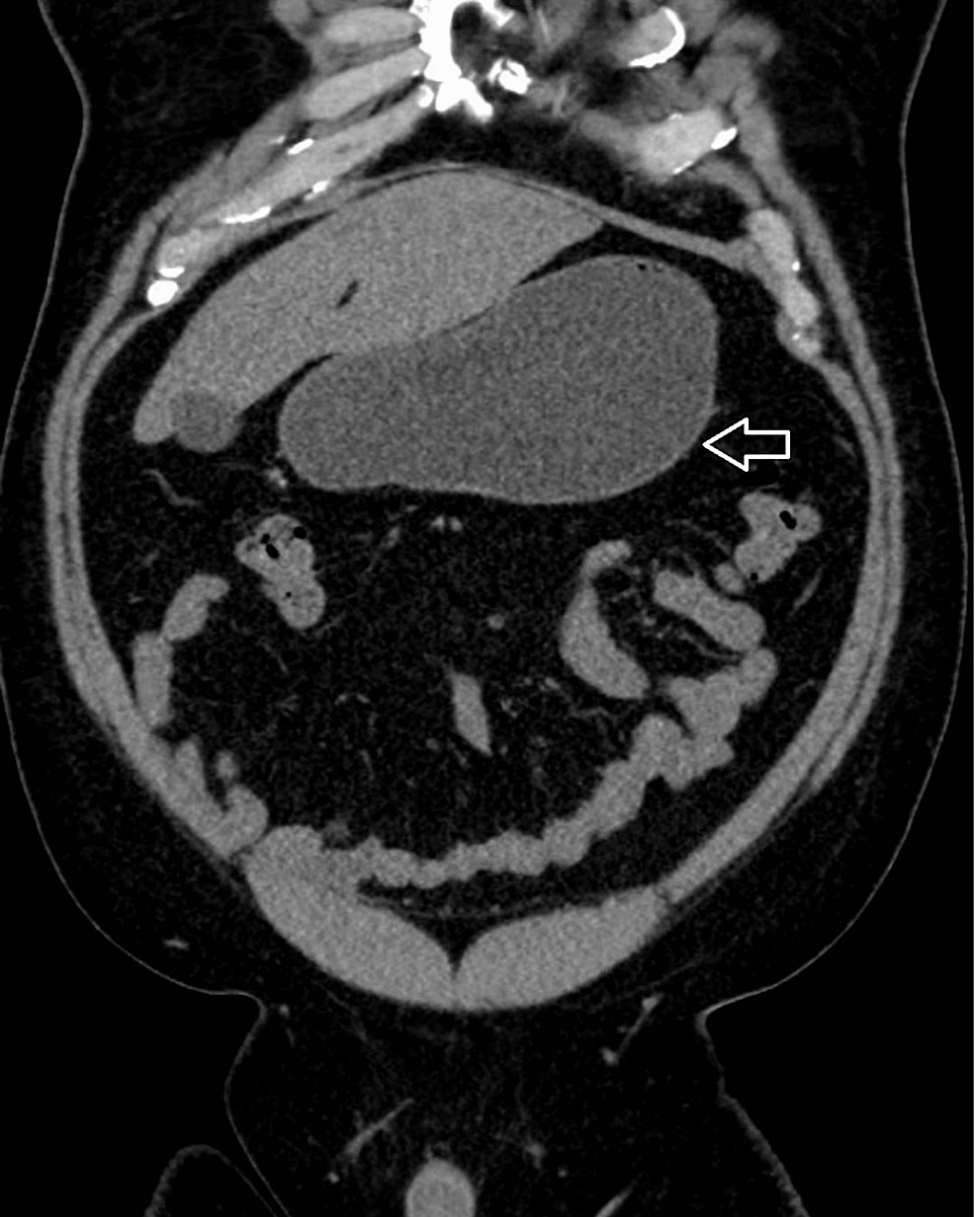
CT abdomen pelvis coronal view showing stomach distention (arrow)
